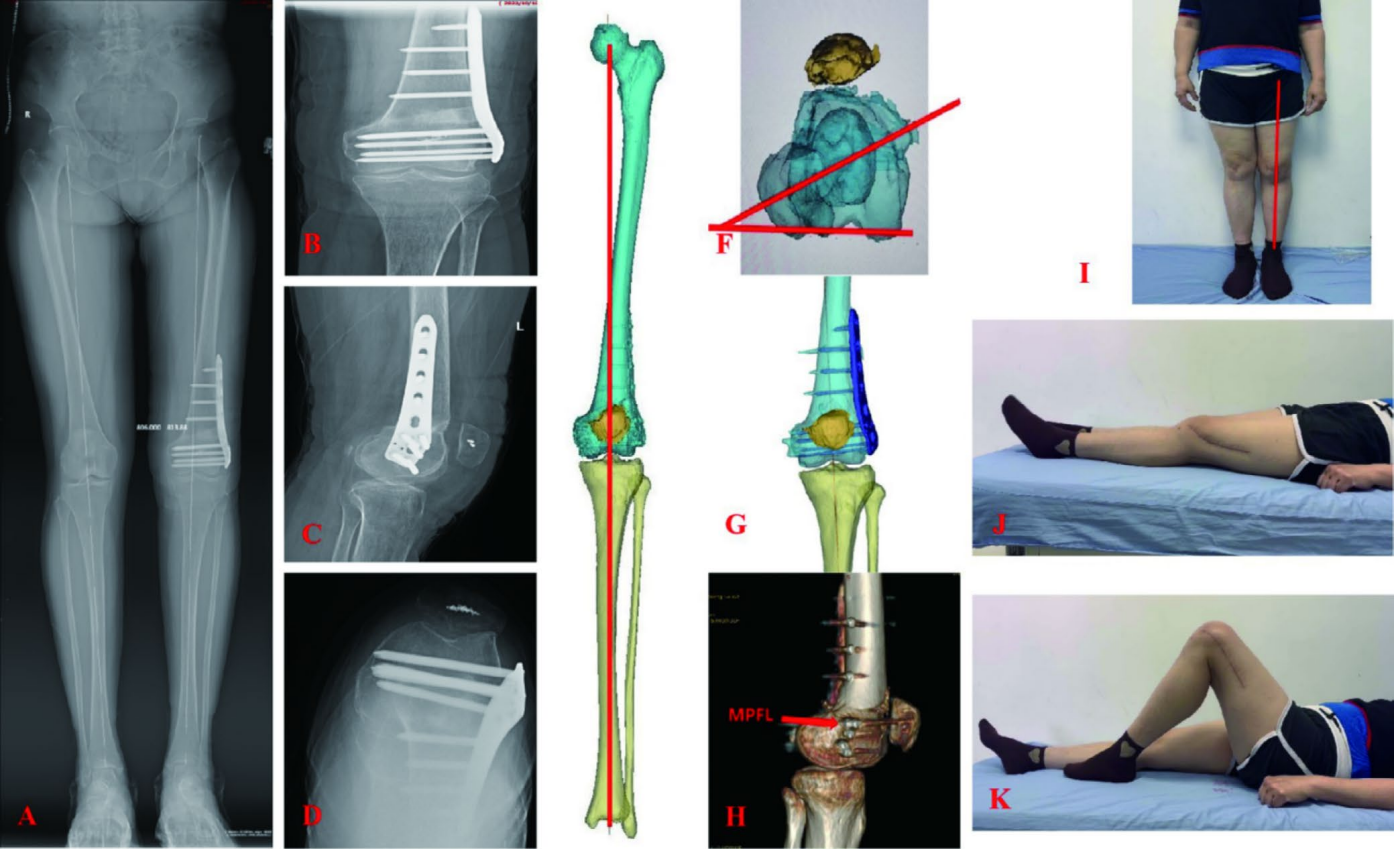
Postoperative photographs and fluoroscopy. (A) The postoperative standing X‐ray of the lower limbs. (B) The postoperative X‐ray of the knee in anteroposterior position. (C) The postoperative X‐ray of the knee in lateral position. (D) The postoperative X‐ray of the knee in 90° axial position. (E) The postoperative 3D‐CT showed that the lower limb alignment returned to normal. (F) The postoperative 3D‐CT showed that the femoral anteversion angle was 15°. (G) The postoperative 3D‐CT showed that the patellofemoral relationship returned to normal. (H) The postoperative 3D‐CT indicated that the femoral stop position of reconstructed MPFL was satisfactory. (I) Good function while standing. (J) Good function while knee extension. (K) Good function while knee flexion.
